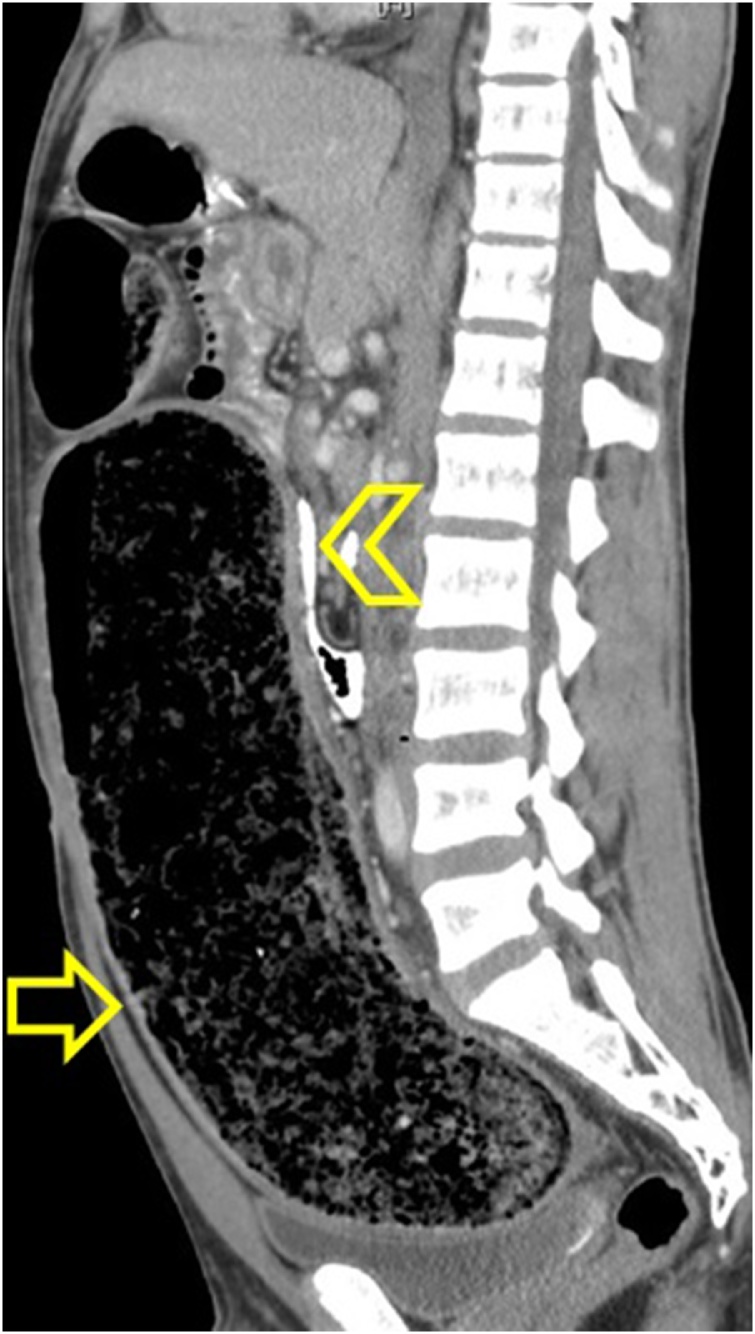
CT scan sagittal view showing a distended sigmoid colon (arrow) and a compressed bowel loops (arrow head).
